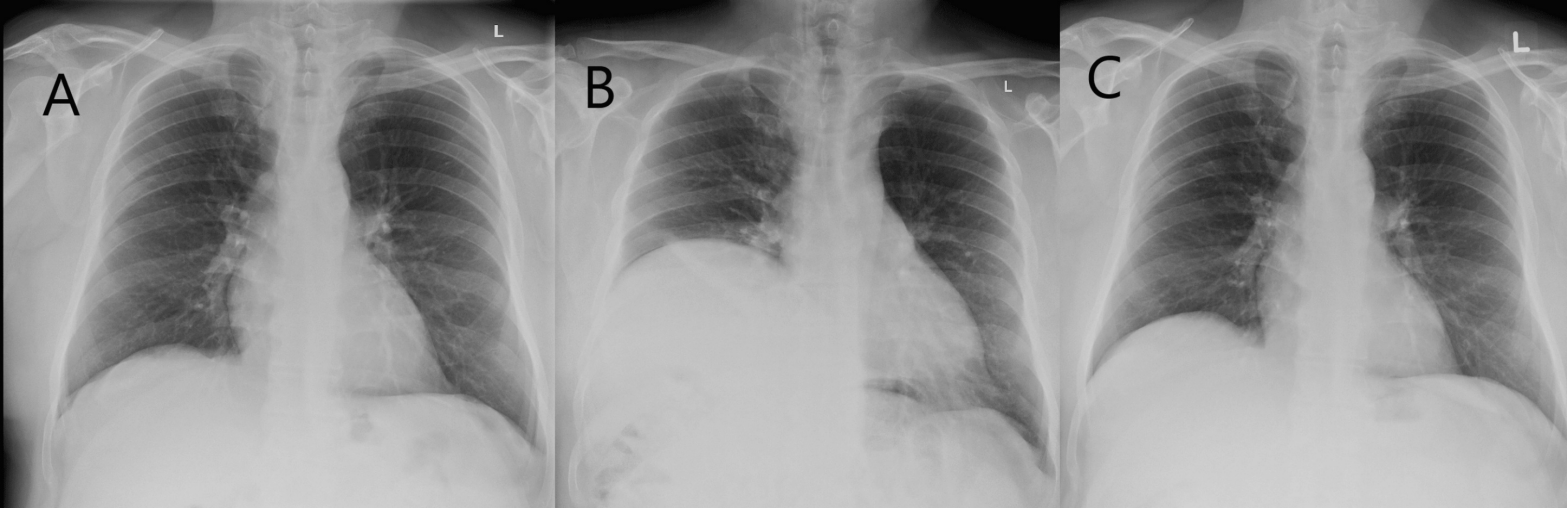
CXRs before and after the procedure using interscalene local anesthesia. (A) Normal preoperative CXR. (B) Day 1 postoperative, showing grossly elevated hemidiaphragm. (C) Day 3 postoperative, showing the paralysis having been resolved and the hemidiaphragm returned to a normal position CXR: chest X-ray
